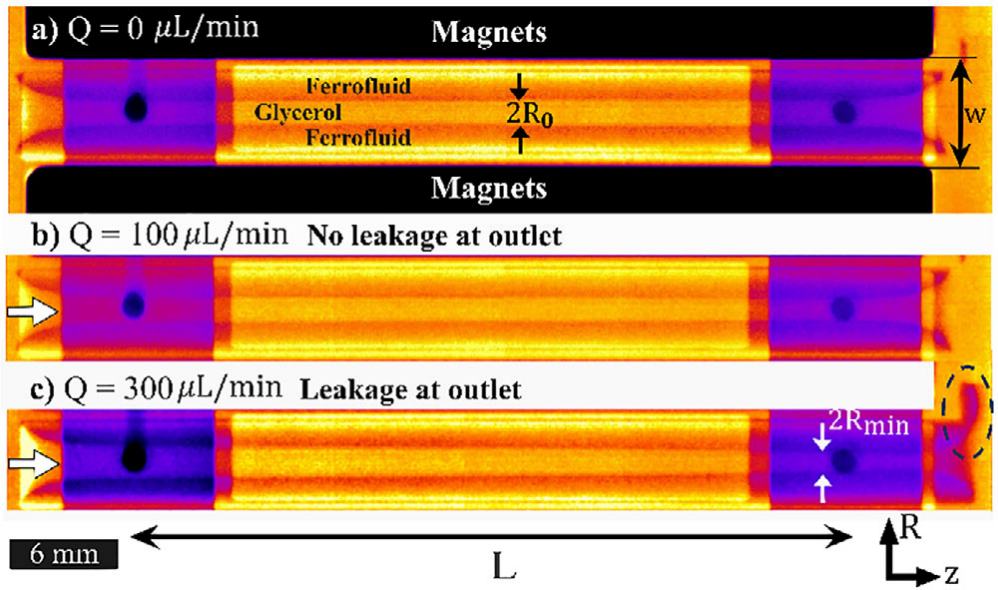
X‐ray radiography of magnetically encapsulated cylindrical flow channels. Glycerol is the inner flowing liquid and APG1141 is the surrounding ferrofluid. a) No flow case *Q* = 0 µL/min with 2*R*
